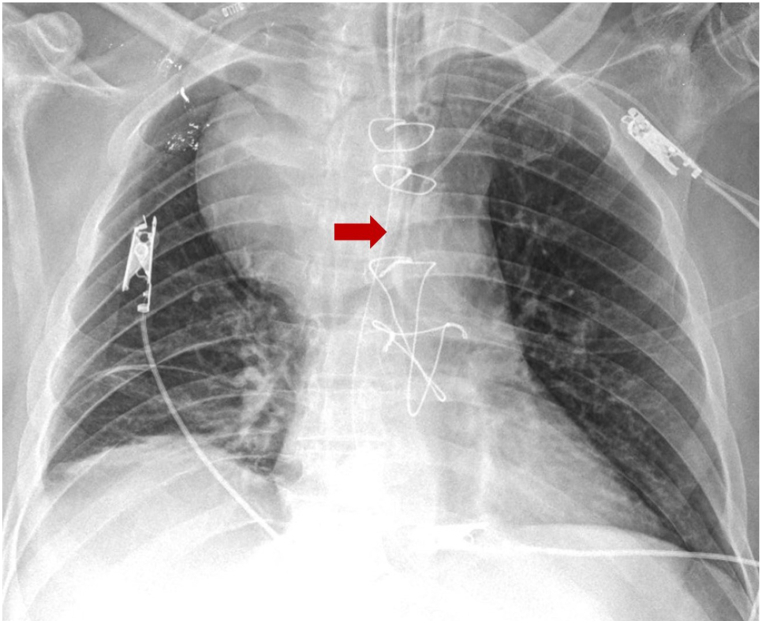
Antero-posterior chest x-ray: Giant aneurysm of the brachiocephalic trunk that conditions severe extrinsic compression of the distal trachea (red arrow). Orotracheal tube in proximal trachea.
